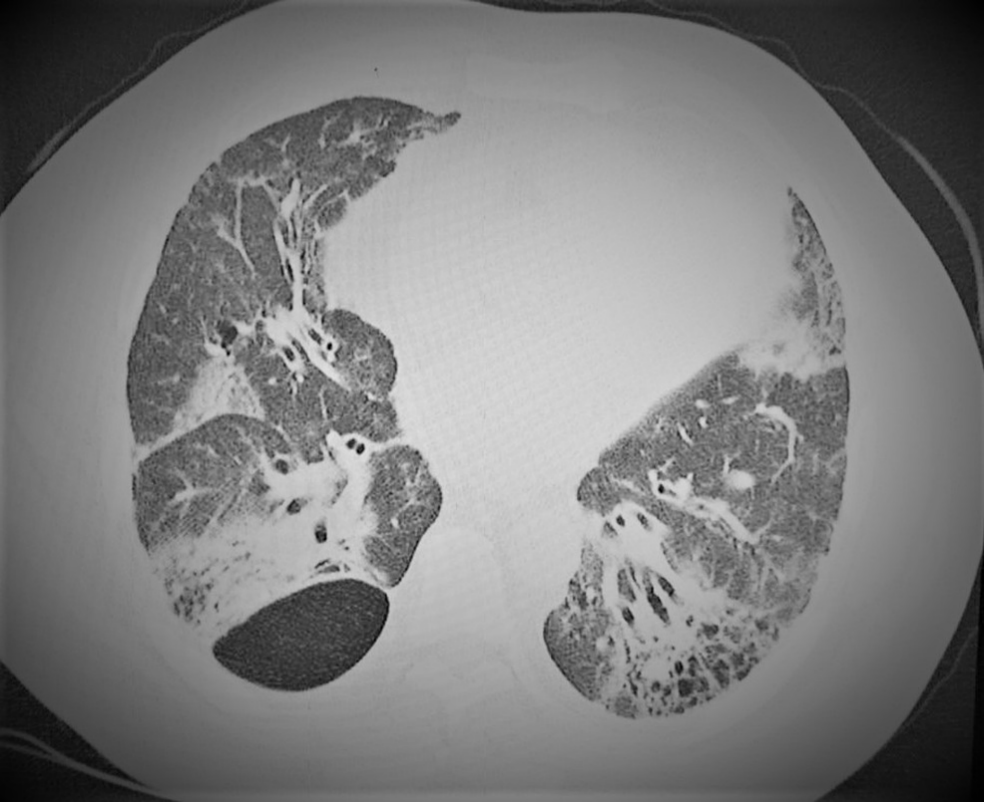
Chest CT scan: lung parenchyma window showing architectural distortion with bronchiectasis, bilateral apical and basal honeycombing pattern with diffuse perilobular septal thickening, and the presence of diffuse perilobular bilateral basal infiltrates.
